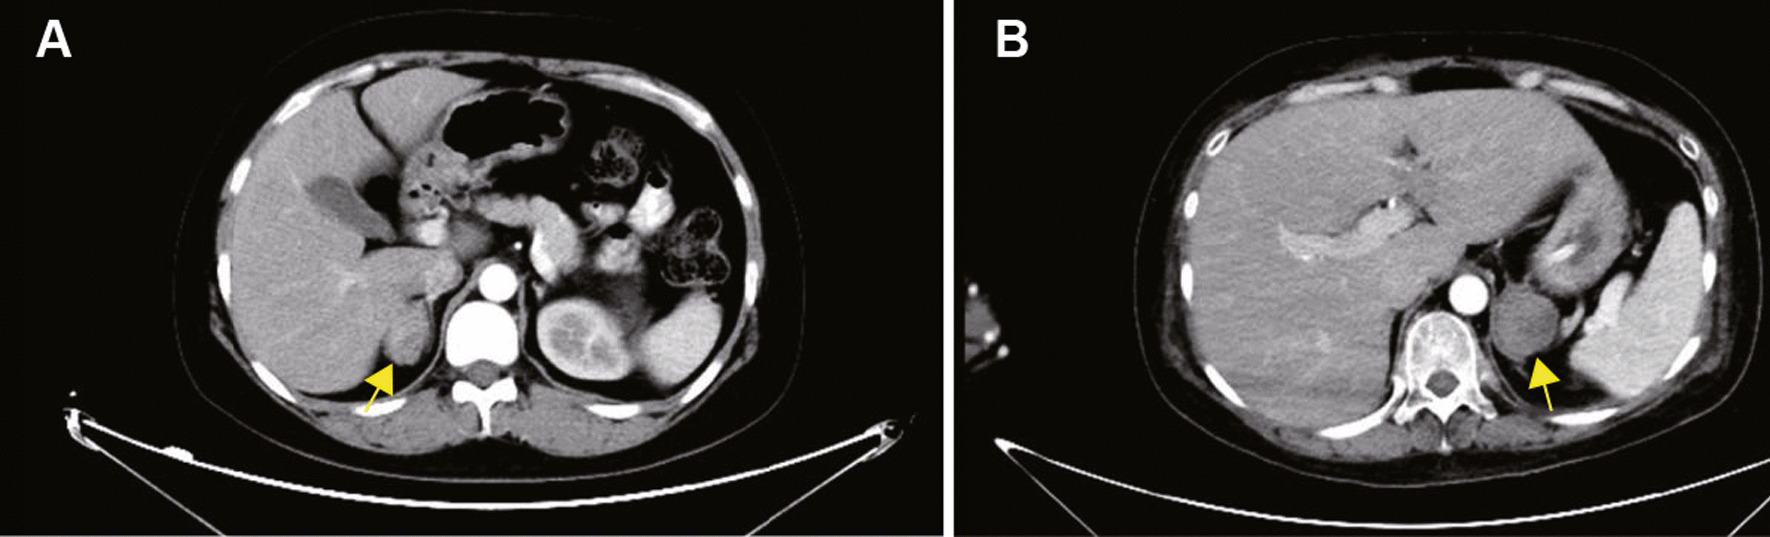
Representative adrenal CT images of non-pregnant and pregnant patients. A Adrenal CT image of non-pregnant patient with CPA. B Adrenal CT image of pregnant patient with CPA. The adenoma was marked by yellow arrow
